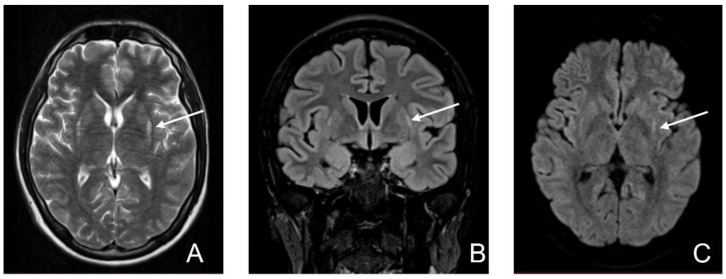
Fourth MRI (D45). (A) T2-wi sequence: limited hyper signal in bilateral putamen in the posterior external region; (B) Cor FLAIR image: minimal hyperintensity in the periphery of bilateral putamen; (C) Axial DWI: minimal restricted diffusion persistent in the periphery of bilateral putamen (white arrow).
